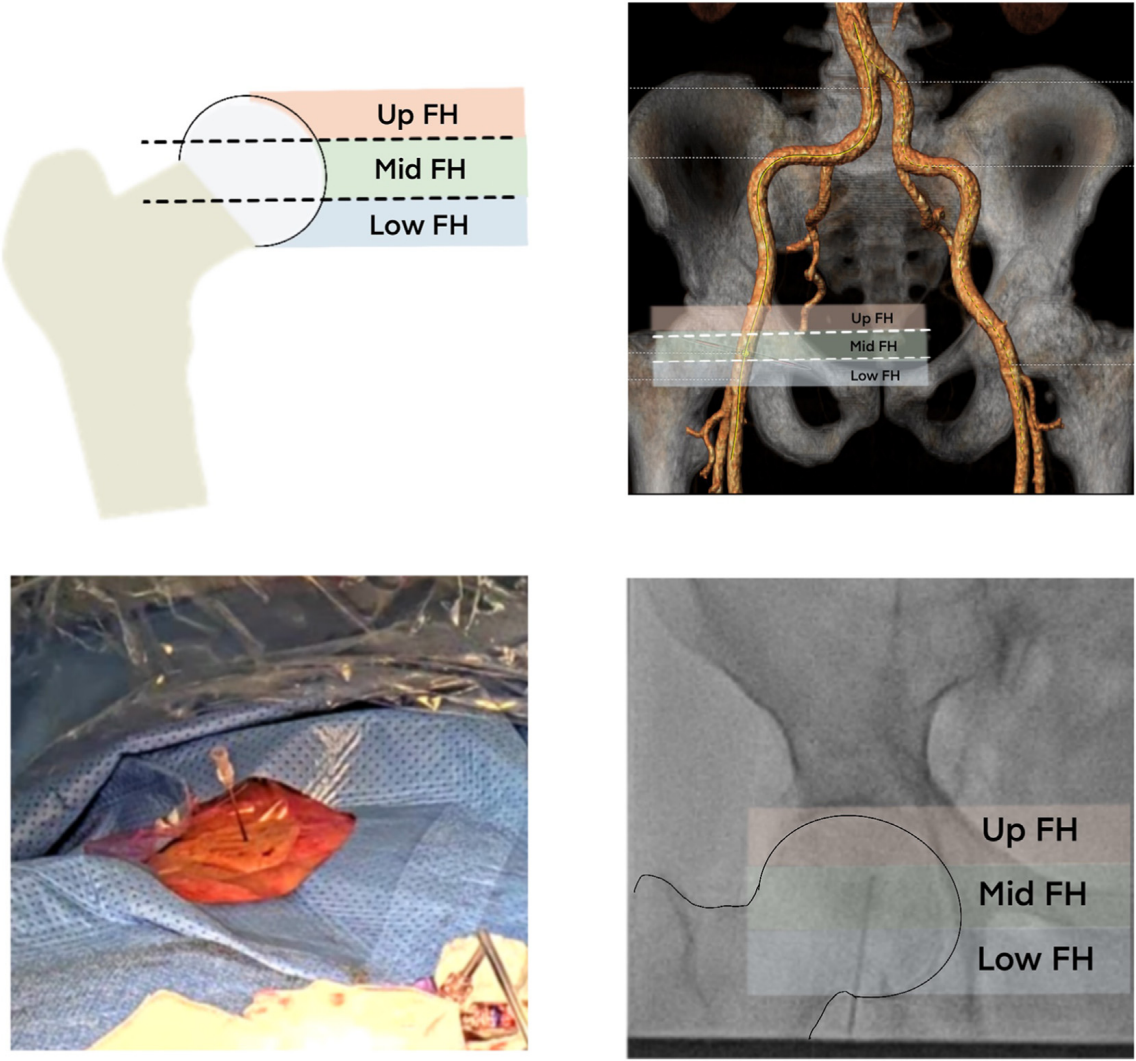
Integrated evaluation of the puncture site in computed tomography and fluoroscopy. The femoral head was divided into the upper, middle, and lower thirds. The paralleled three segments of femoral artery are the three puncture candidate sites. A 45-degree puncture needle marks the location under fluoroscopy and aims the predefined puncture site. Abbreviation: FH, femoral head.
